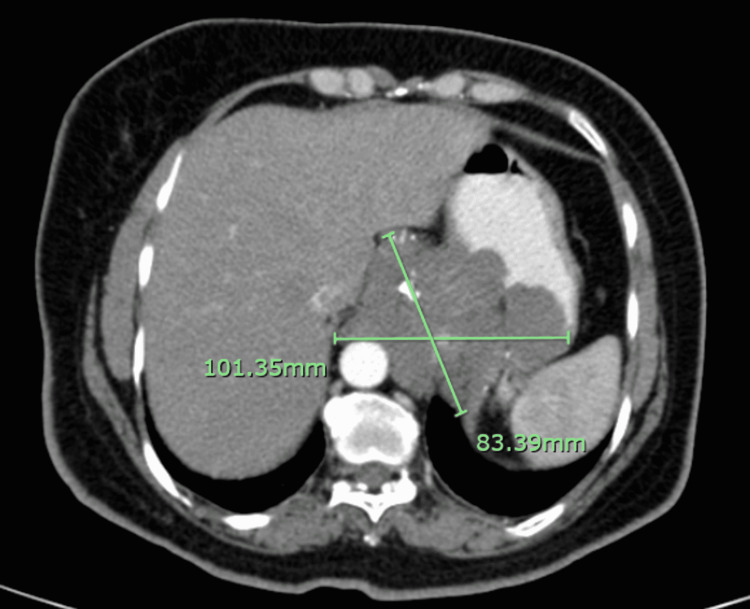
Computed tomography image showing the hemangioma before the coil embolization therapy
